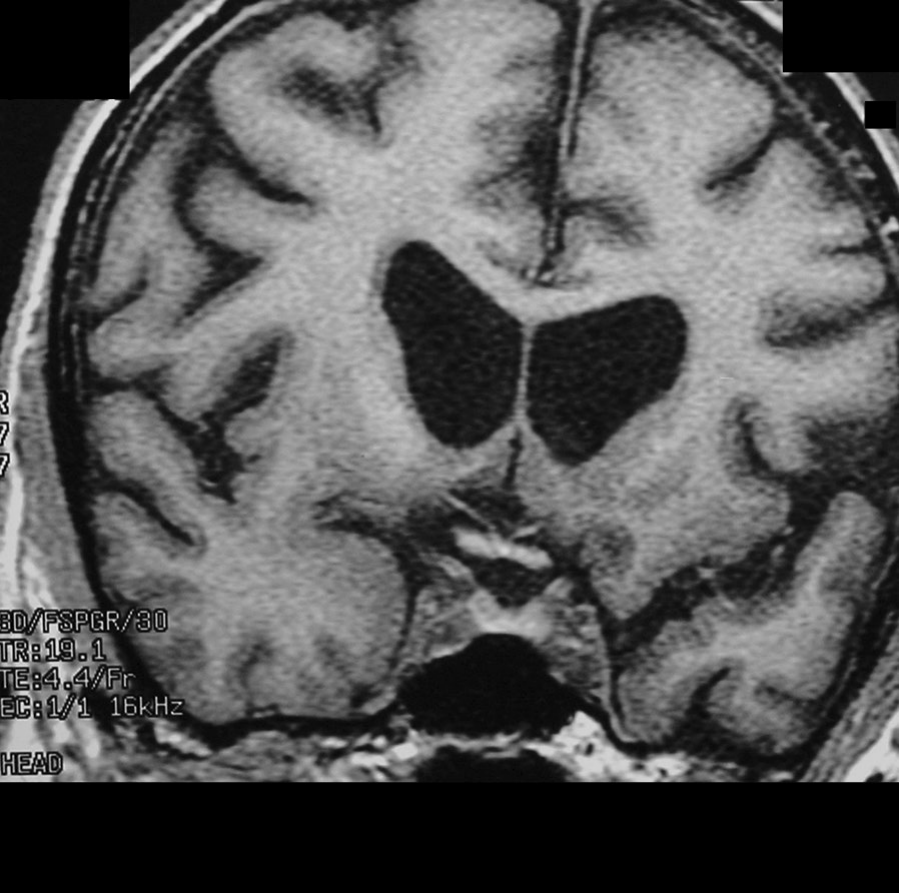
Coronal T1-weighted image demonstrates atrophy of the bilateral caudate heads with “box-shaped” ventricles
